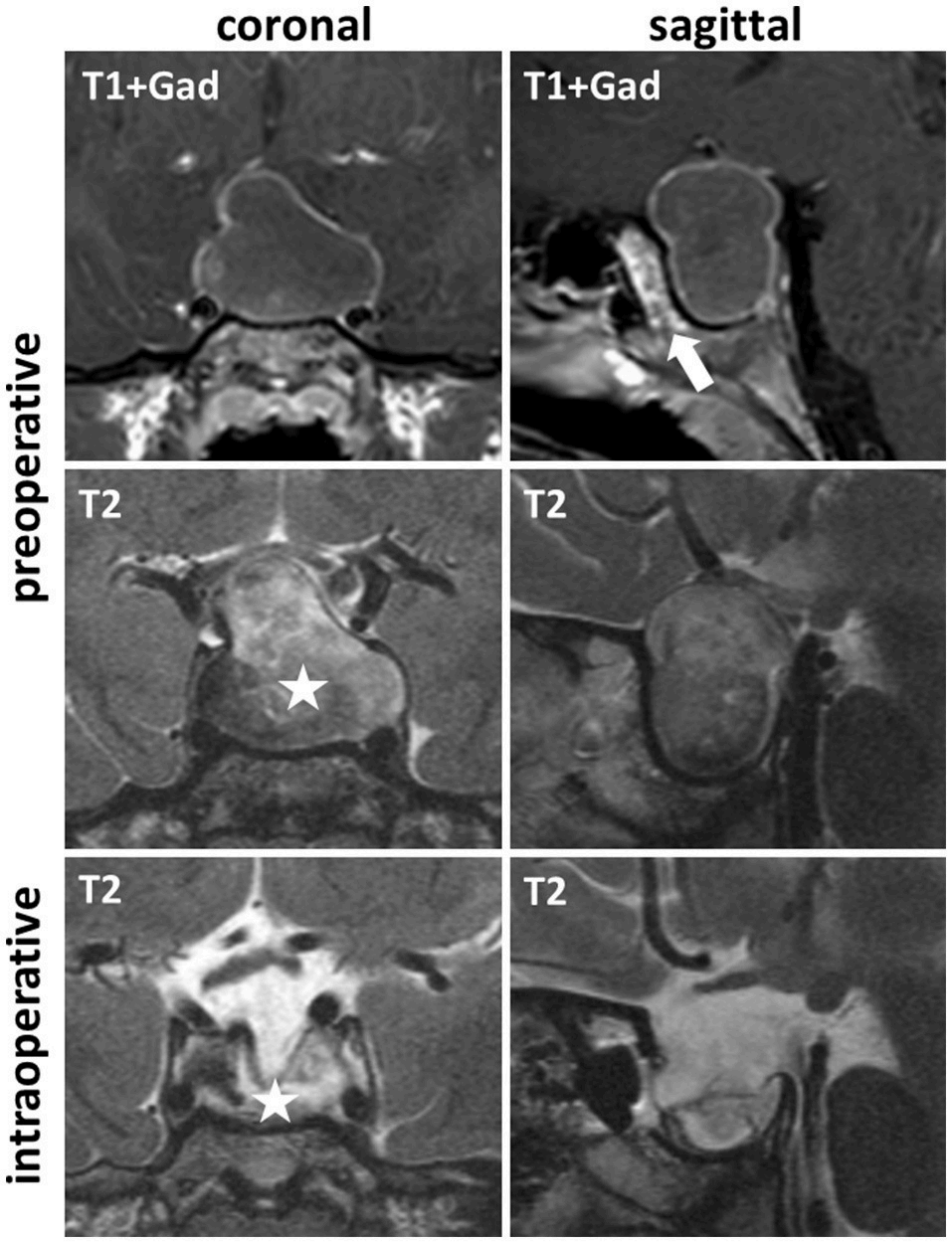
Pre- and intraoperative MRI; T1-weighted MR images after gadolinium contrast medium application (T1+Gad) and T2-weighted MRI showed in coronal and sagittal orientation a preoperative intra and suprasellar cystic tumor, lacking sphenoid sinus pneumatization (T1+Gad, sagittal, arrow). Intraoperative imaging confirmed complete drainage of the cyst (T2 coronal, asterisk).
